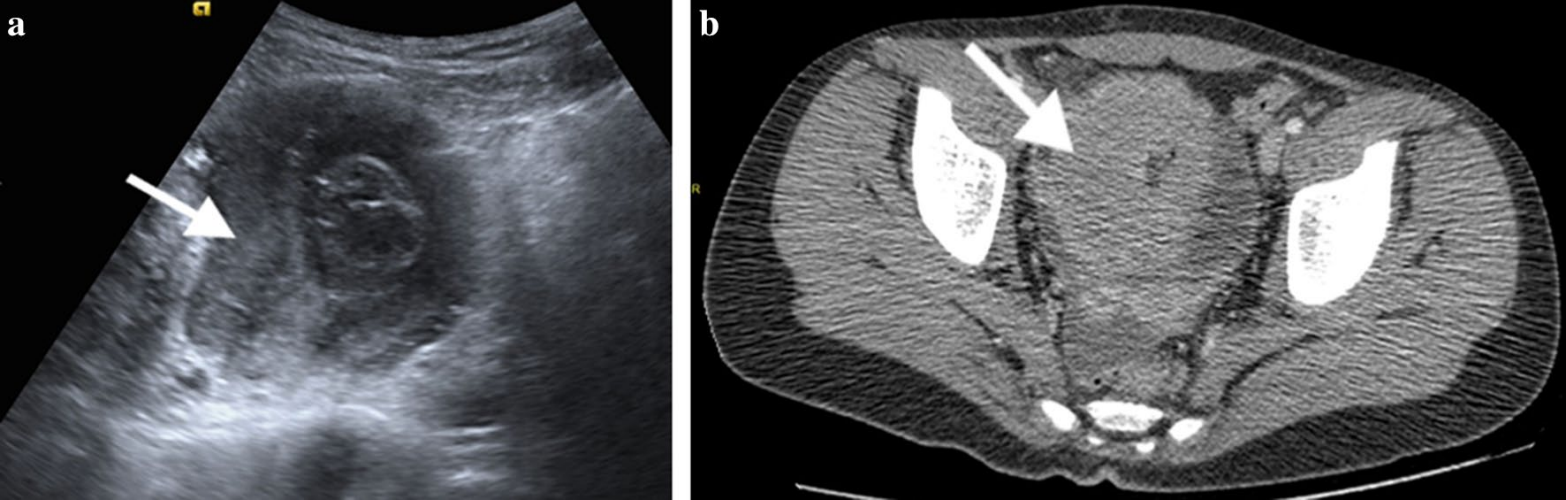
a, b A 11-year-old girl with Burkitt lymphoma. She complained of abdominal pain for the last 2 months. a US image demonstrates pathological diffuse thickening of the intestinal wall (arrow) causing intussusception at the level of the ileal loops and appearance of a pseudo-mass. b Axial post-contrast CT image shows mass lesion (arrow) in the right lower quadrant in the intestinal wall. Also note the pelvic fluid
